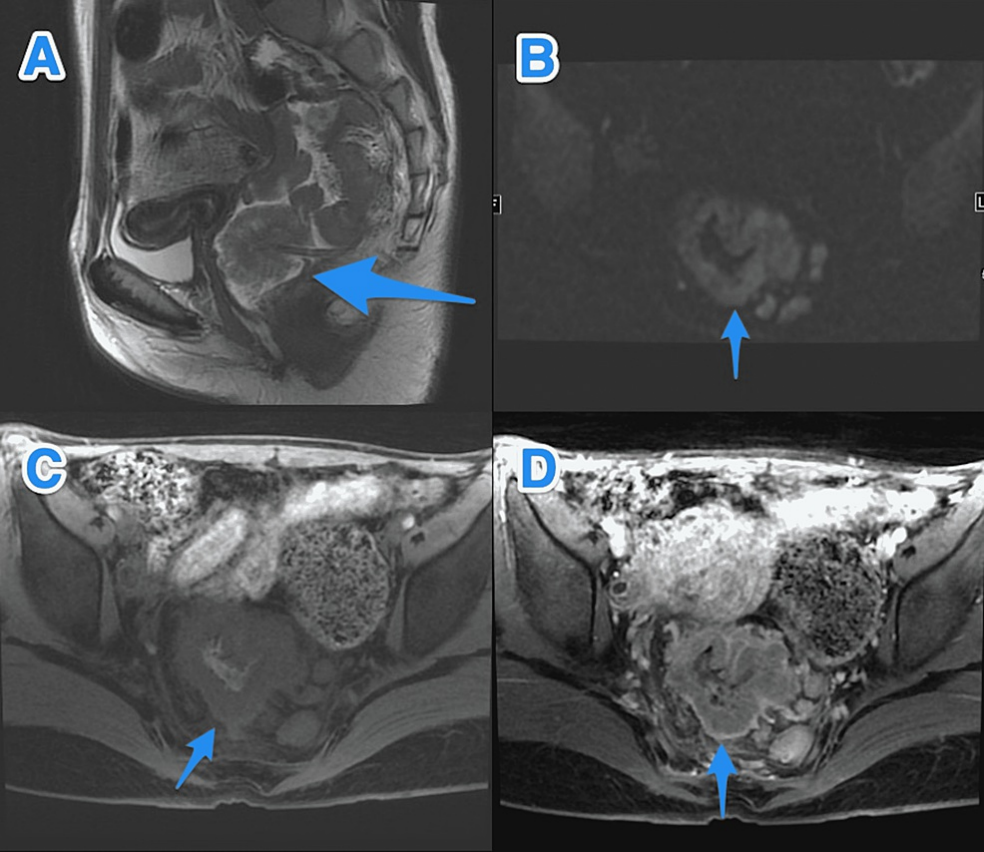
Baseline MRI study of the pelvis. MRI study of the pelvis sagittal T2 (A), axial DW (B), axial T1 (C), and axial T1 post-contrast (D) photographs show mid rectal circumferential wall thickening (small arrow) measuring 6.5 cm in length and 3.5 cm from the anorectal junction. This mass is invading the mesorectal fat at the left postero-lateral aspect with multiple large regional lymph nodes. Another large pedunculated polyp is seen in the lower rectum (large arrow), 2.2 cm from the anorectal junction with stalk arising from the posterior rectal wall. DW: diffusion-weighted
